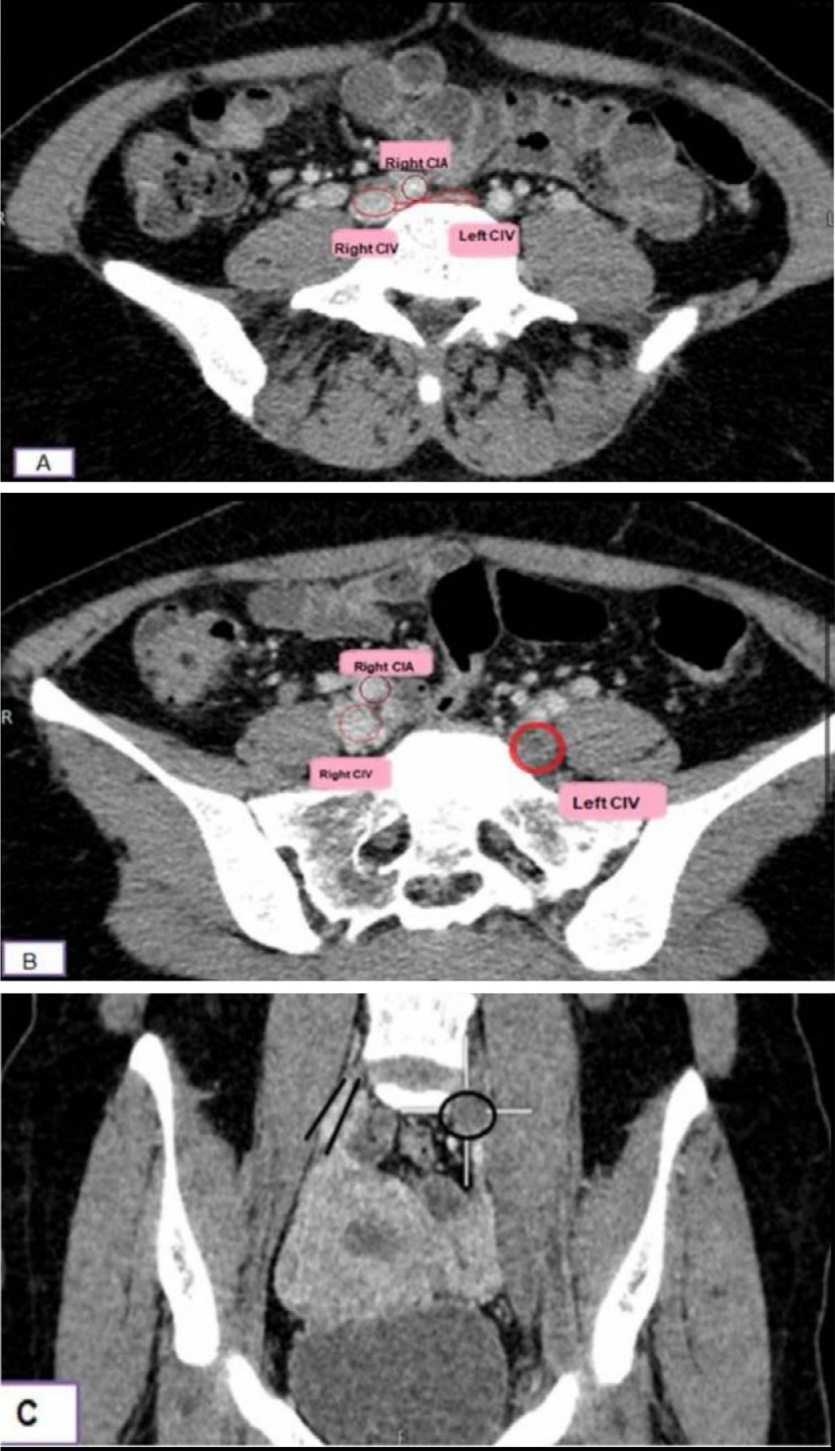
CT Porto-venous phase axial (A, B) and coronal (C). Views: There is compression of the left common iliac vein by the overlying right common iliac artery consistent with May-Thurner syndrome (image A). Filling defect is noted within the dilated, nonenhancing left common iliac vein inferring thrombosis compared to right common iliac vein (image B and C).
